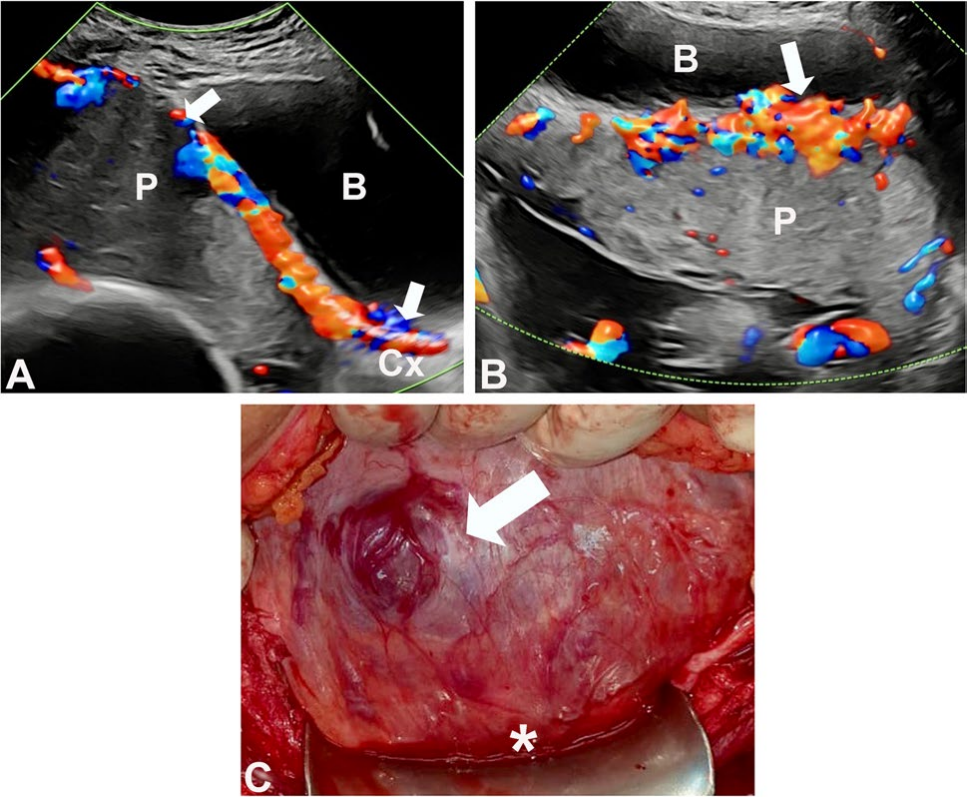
Case of a patient at 36 weeks with no evidence of PAS at birth. A: Longitudinal TAS CDI mapping of the LUS showing a placenta (P) previa partially covering the cervix (Cx) and showing increased uteroplacental vascularity along the utero-bladder interface (between arrows); B: Transverse TAS CDI mapping showed an area of focal merged subplacental uterovesical hypervascularity interconnected with bridging vessels (arrow) corresponding to a rail sign.; C: Intraoperative view of the anterior LUS wall at laparotomy before dissection of the bladder (*) showing a small area of enlarged subserosal vessels (arrow) corresponding anatomically to the ultrasound area (arrow) in B. P= Placenta; B= Bladder
